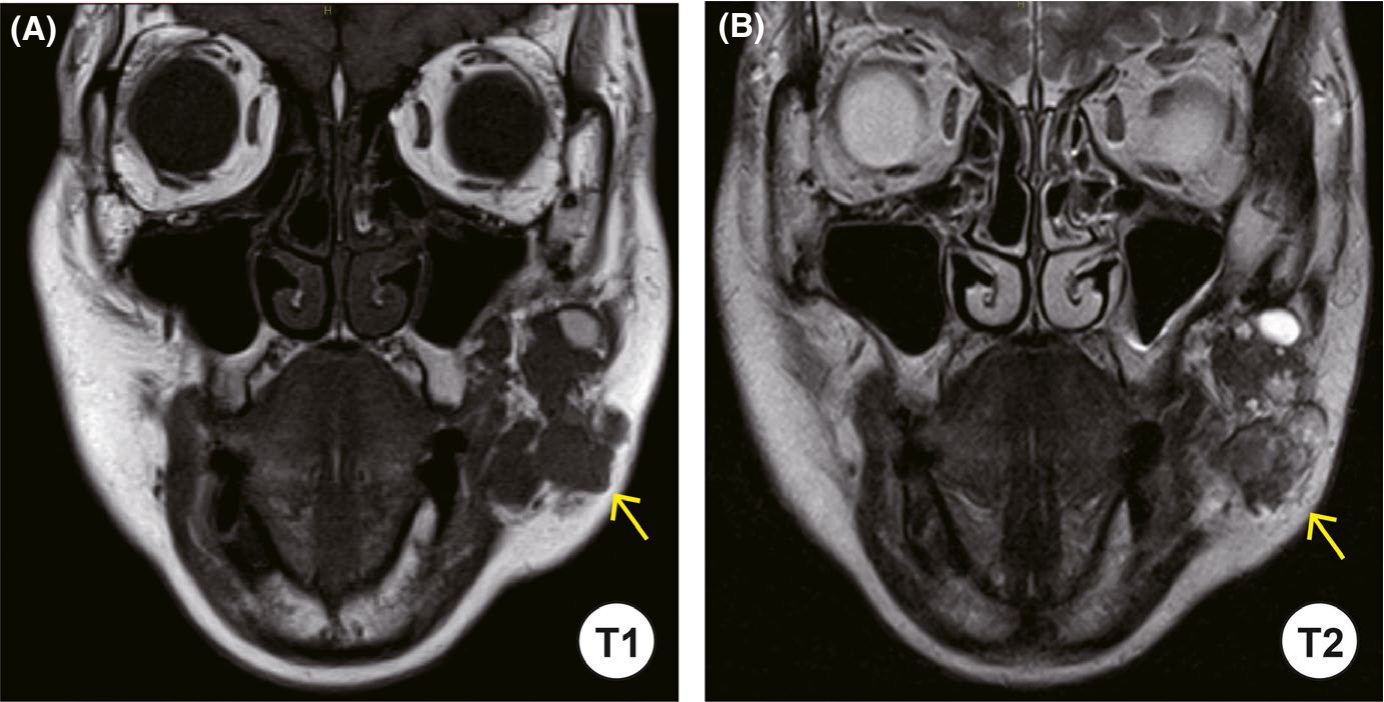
T1‐ and T2‐weighted MRI of the tumor masses. MRI showed low‐intensity nodules on T1‐weighted (A) and heterogeneous nodules with mixed high‐ and low‐intensity signals on T2‐weighted (B) images. MRI, magnetic resonance imaging
